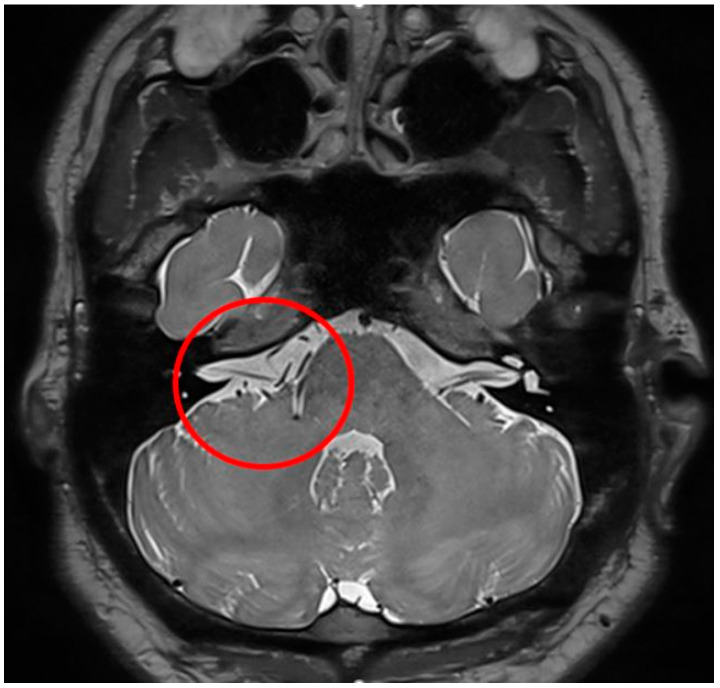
A 34-year-old man with right HFS, shown in a preoperative proton density-weighted (PD-weighted) magnetic resonance image (MRI). At the exit region of the right facial nerve root, the right anterior inferior cerebellar artery (AICA) and posterior inferior cerebellar artery (PICA) have a complex appearance (red circle).
